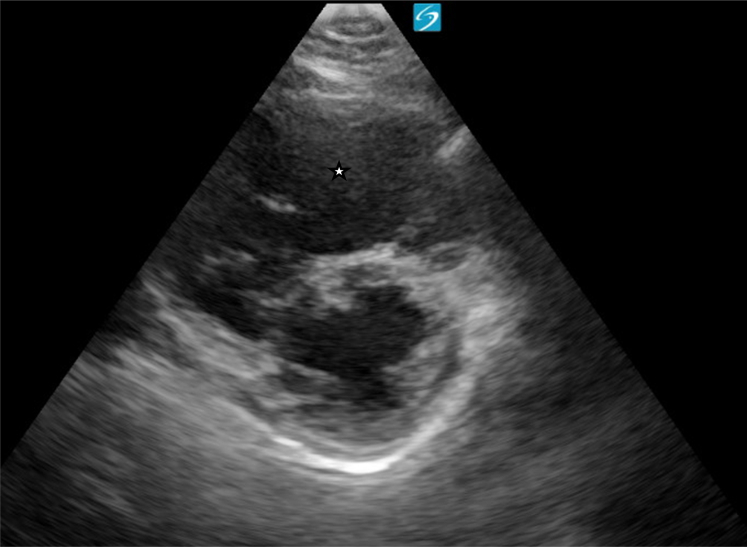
Point-of-care ultrasound (parasternal short-axis view) showing right ventricular dilation. Image taken by the author.
